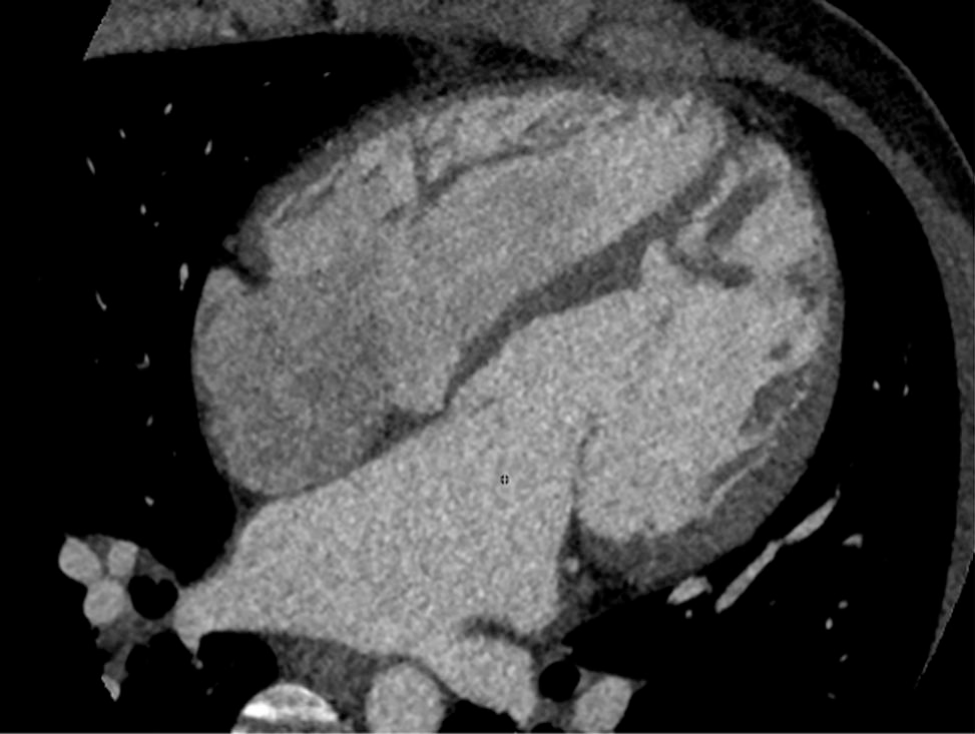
Contrast-enhanced CT image, apical four-chamber plane showing atrioventricular discordance and dilatation of both ventricular cavities.
